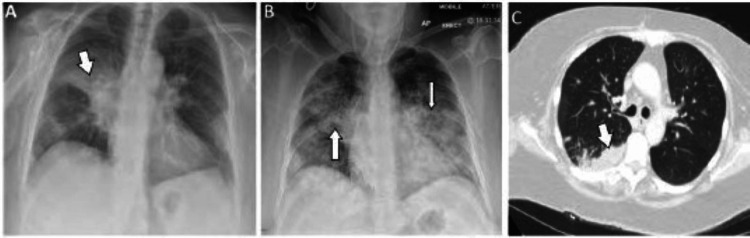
(A) Chest radiograph antero-posterior (AP) projection, showing right mid-zone heterogeneous opacity. (B) Chest radiograph AP projection showing diffuse bilateral heterogeneous opacities (white arrows). (C) Computed tomography scan of chest showing ill-defined patchy consolidation more prominent on the right side.
